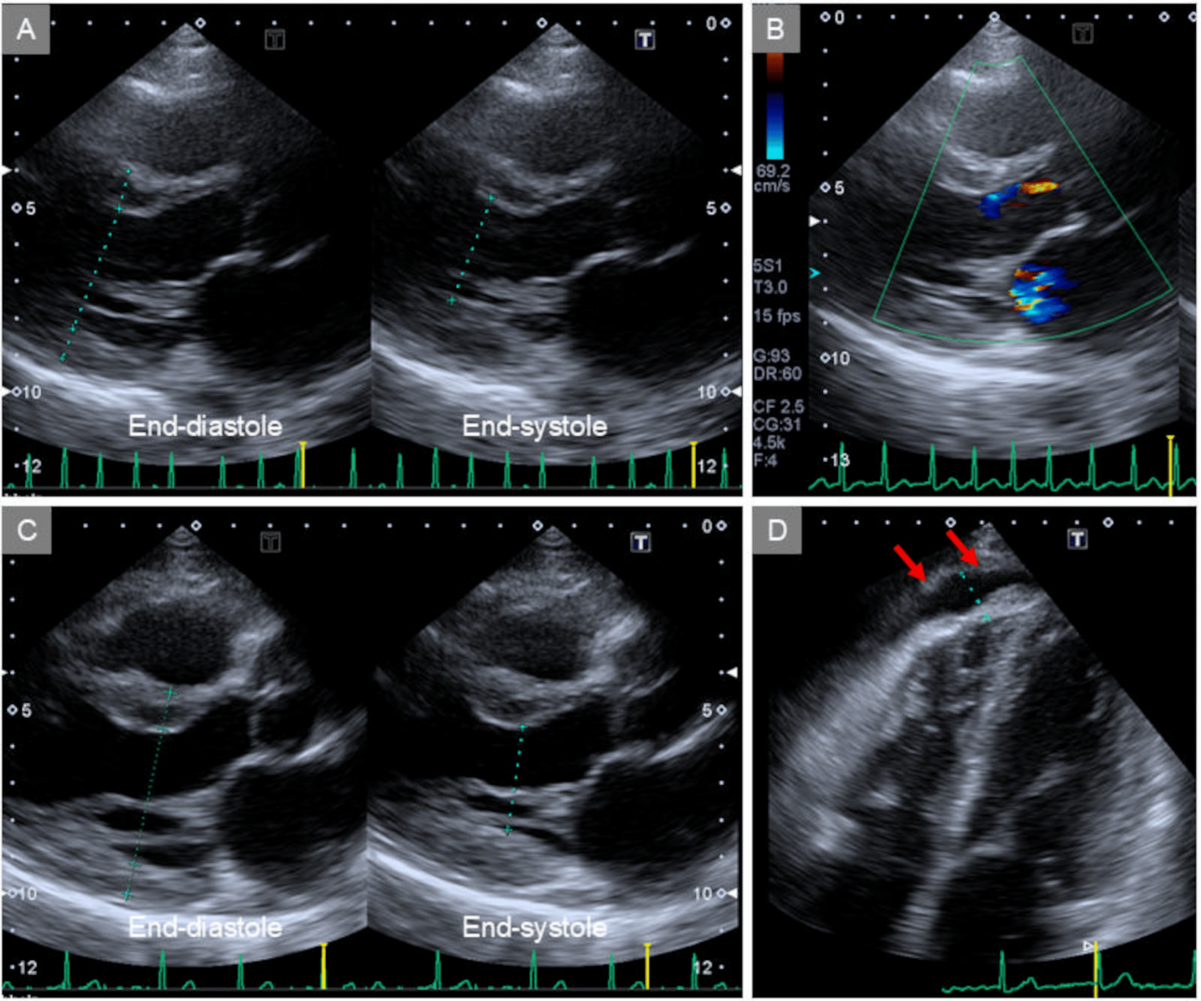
Echocardiographic images at presentation and on hospital day 7 At presentation, transthoracic echocardiography revealed globally reduced LV systolic function, with an EF of approximately 30% (A). Moderate mitral regurgitation was noted (B). On hospital day 7, repeated echocardiography demonstrated marked improvement in LV function over a short period, with an EF of 60% (C). A small amount of pericardial effusion remained (D, arrows). LV: left ventricular, EF: ejection fraction
